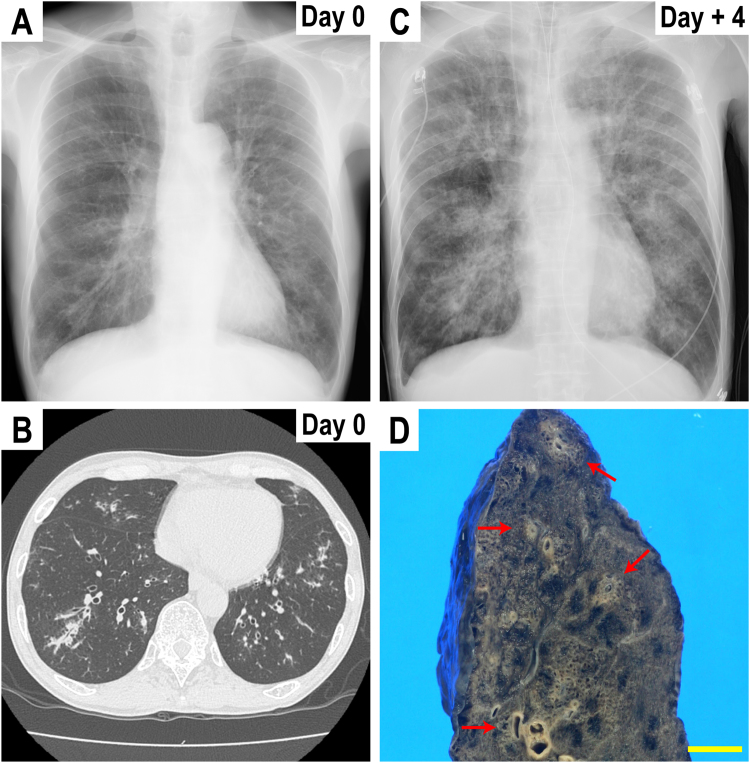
Radiological images and macroscopic findings. (A) Chest radiograph upon admission (Day 0, standing position). (B) Thoracic CT (Day 0) showing a slight thickening of the bronchial walls. (C) Chest radiograph (Day + 4, decubitus position) showing an infiltrative shadow. (D) Macroscopic findings of the lung at autopsy (Day + 4). Most bronchi are surrounded by ocher membranous lesions (arrows). Scale bar, 10 mm.
